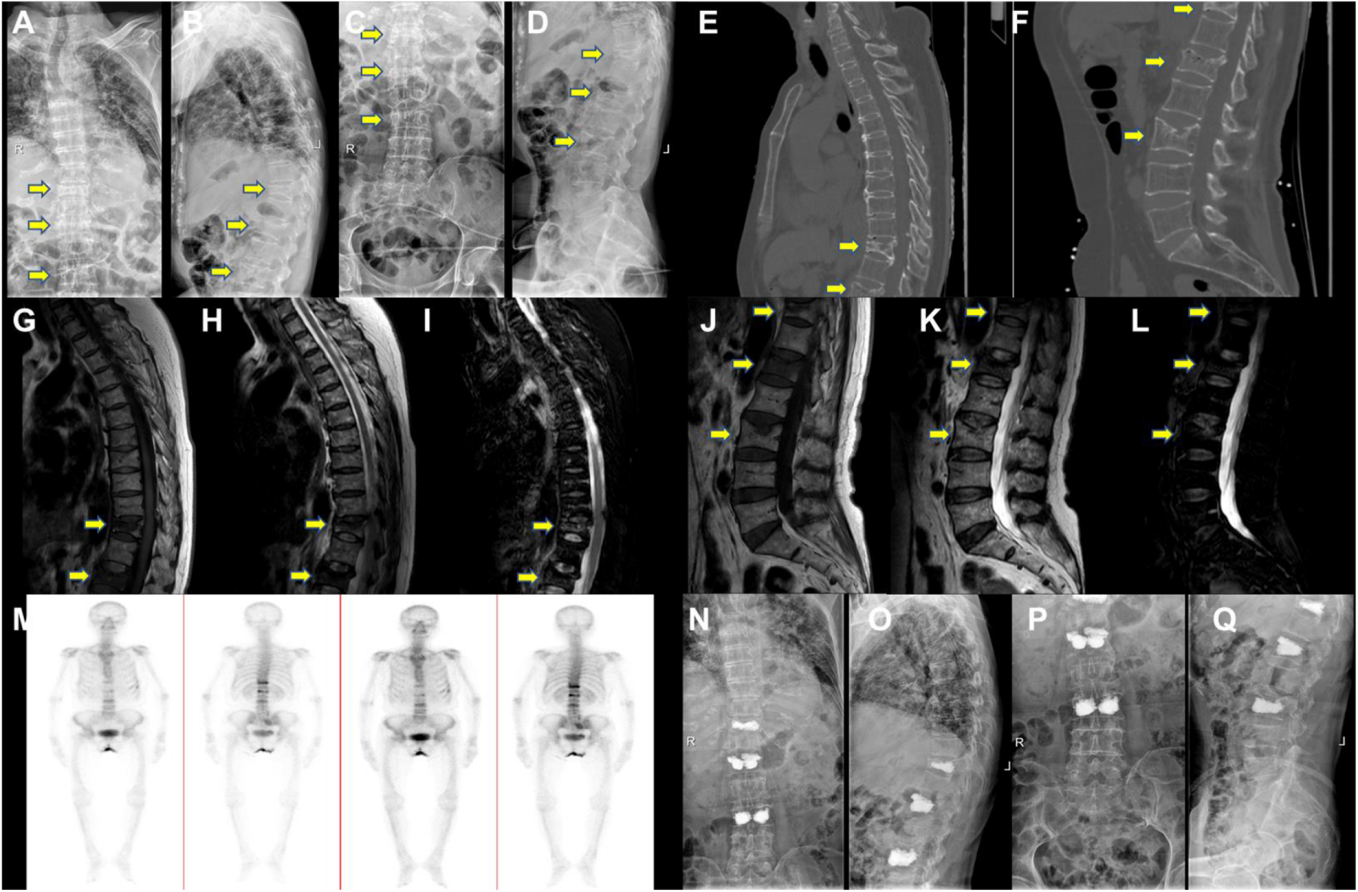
The representative patient's medical images. Pre-Op digital radiography (DR) images of (A,B) thoracic and (C,D) lumbar. Representative CT images of (E) thoracic and (F) lumbar. Representative MRI images of (G) T1, (H) T2, and (I) STIR. Thoracic and (J) T1, (K) T2, (L) STIR lumbar (M) and ECT image. Post-Op DR images of (N,O) thoracic and (P,Q) lumbar. Yellow arrows point to the injured vertebral bodies. ECT, emission computed tomography.
